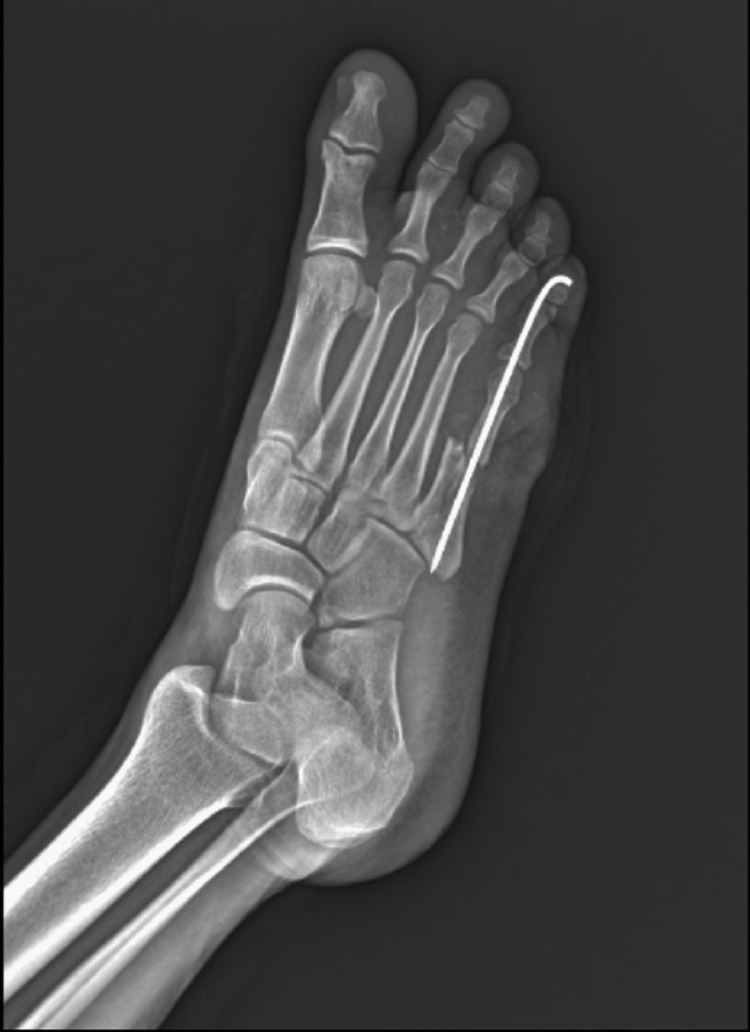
X-ray showing fifth metatarsal fracture managed with K-wire fixation - oblique view
